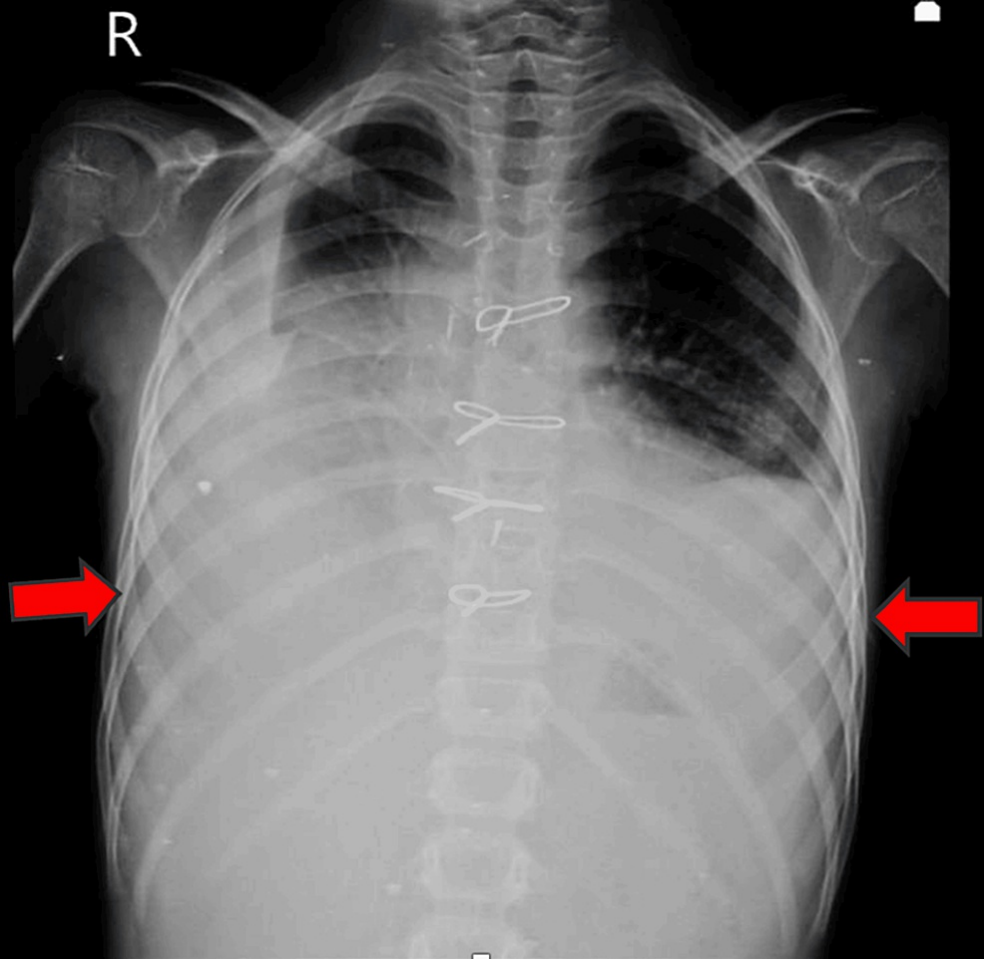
Chest X-ray showing bilateral pleural effusion (red arrows)
